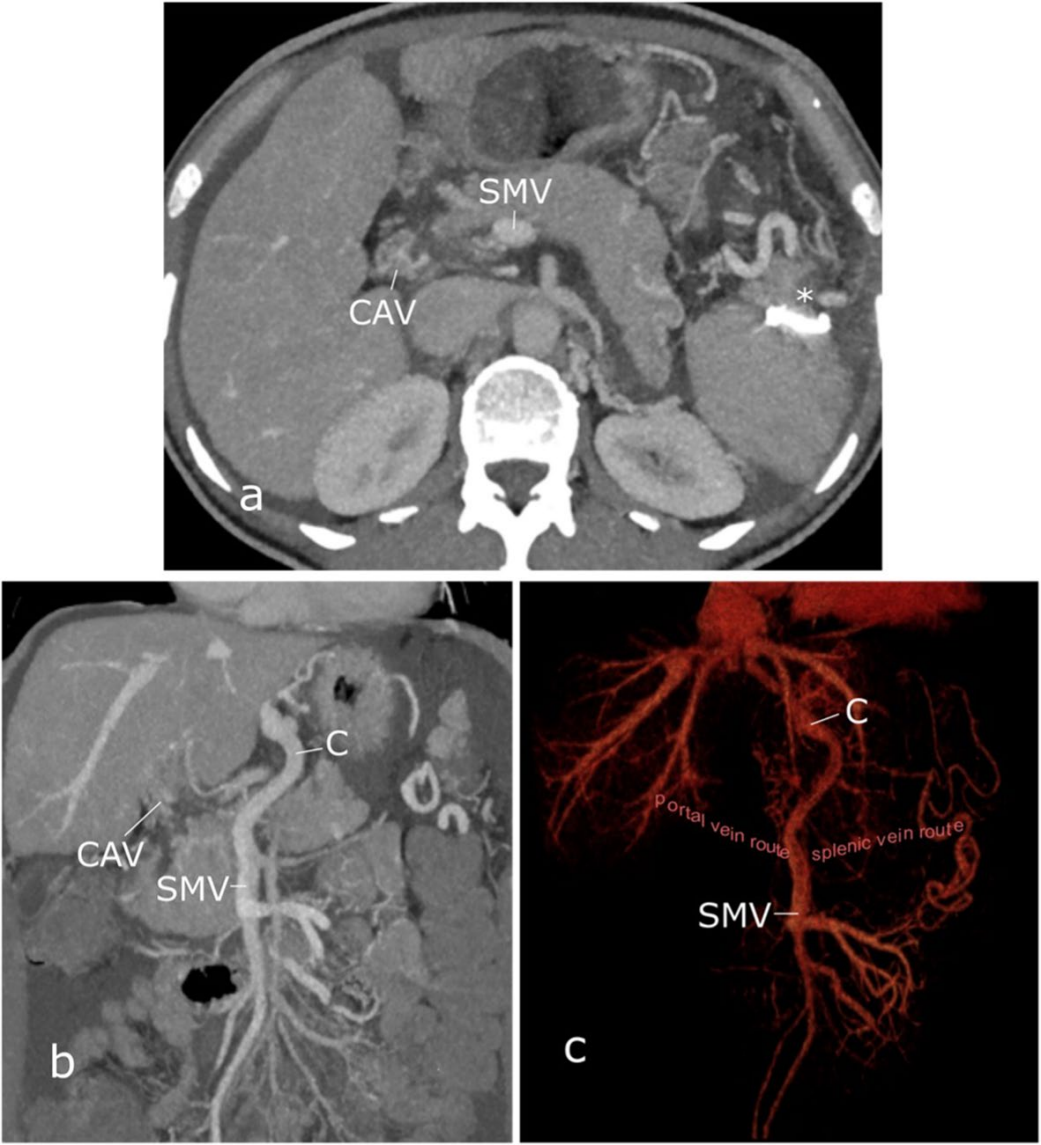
Pre-interventional computed tomography (CT) shows chronic occlusion of the portal vein and splenic vein. Cavernous transformation of the portal vein is evident (CAV). Distinct portosystemic collaterals are seen, especially periesophageally (C). The asterisk marks a coil inserted in the spleen during the previous attempt. a transversal view with maximum intensity projection (5 mm) (b) coronal view with maximum intensity projection (10 mm) (c) coronal view with 3D volume rendering technique reconstruction. The presumed vascular course of the occluded portal vein and splenic vein have been added manually. SMV: superior mesenteric vein
